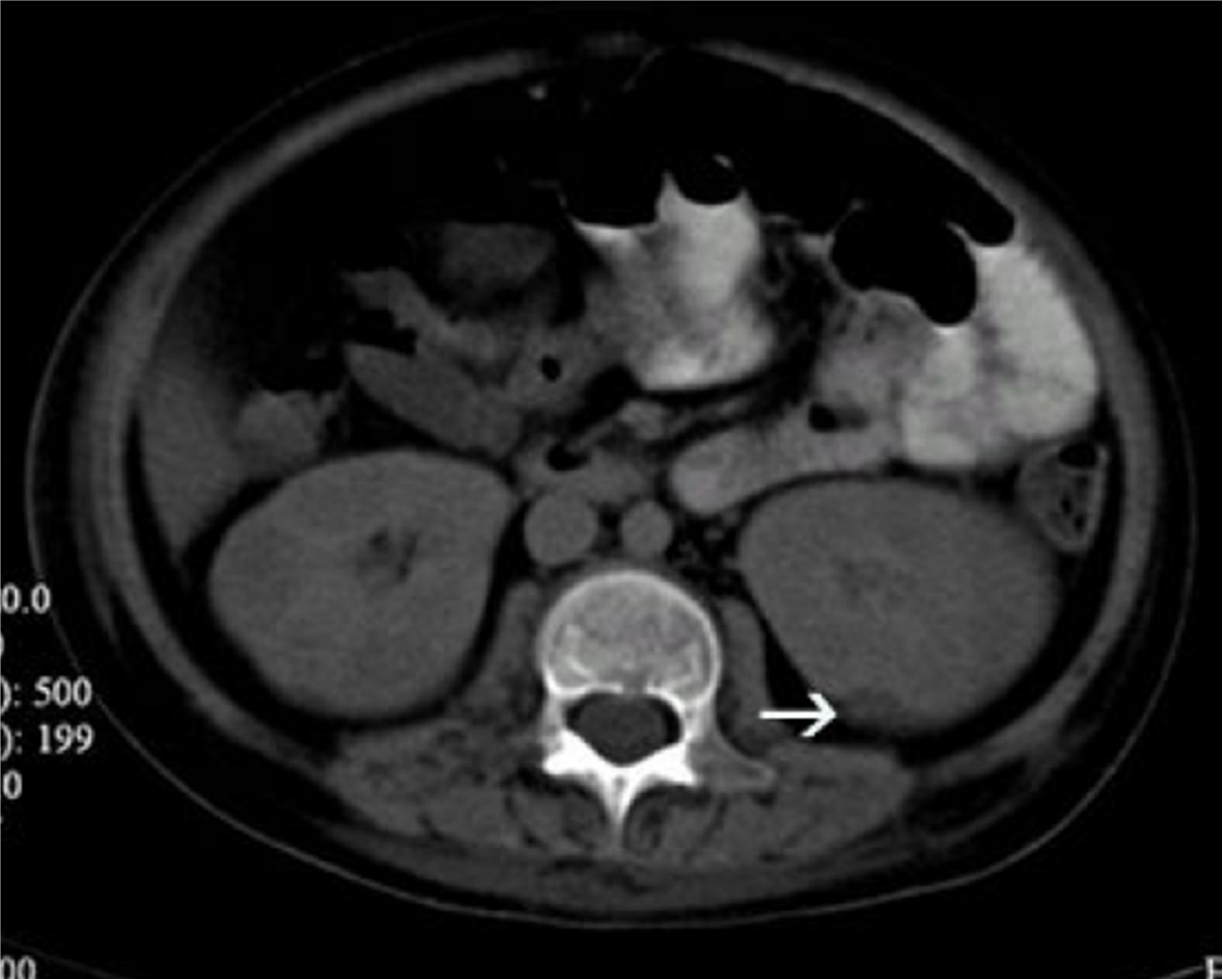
CT abdomen (transverse view). Small hypodense lesion(abscess) in the posterior cortex of the lower pole of the left kidney (white arrow).
